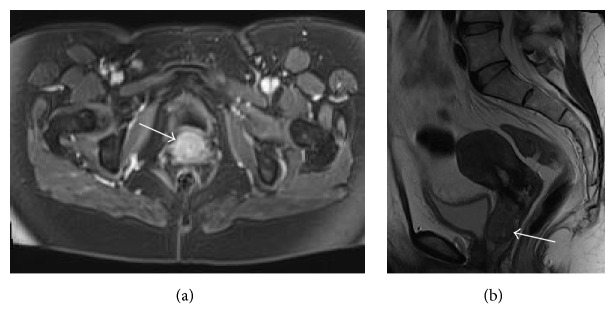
CT scan of the pelvis with (a) and without contrast (b) shows lesion on the anterior wall of the vagina, adjacent to the bladder (denoted by white arrow).
